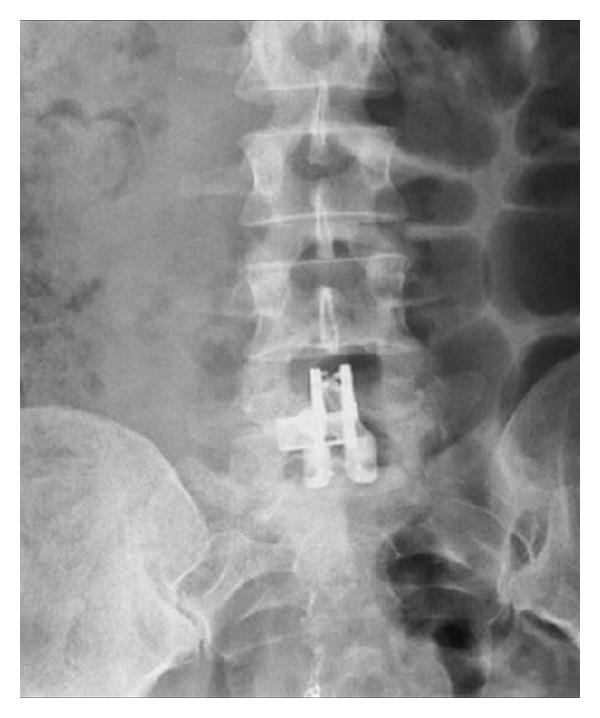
Postoperative anteroposterior X-ray image of Aspen shows the spikes in the lateral plates of the device for bone fixation.
